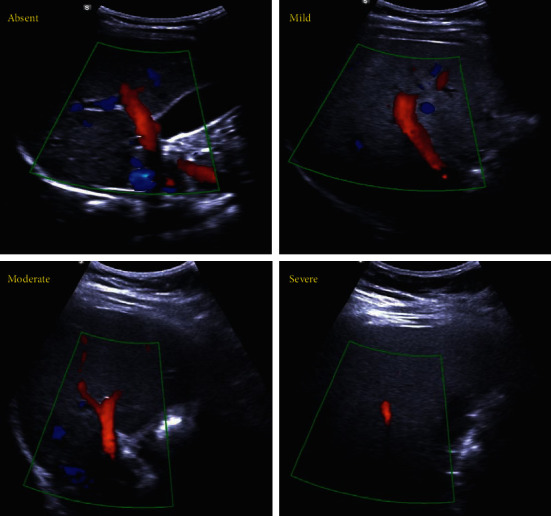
The severity of hepatic fat accumulation assessed by ultrasound.
